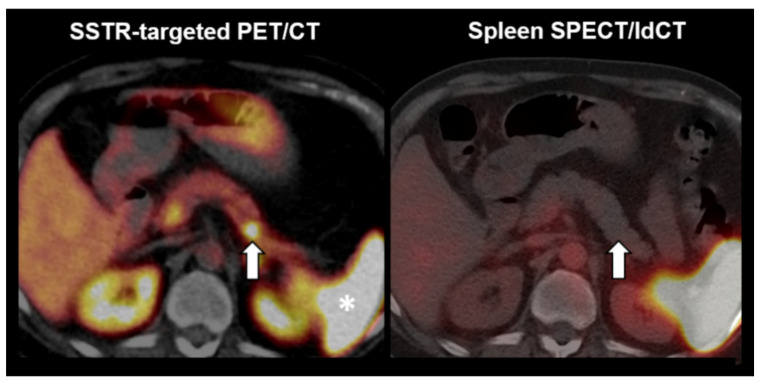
Example of a negative reading in spleen scintigraphy. (Left) Somatostatin receptor (SSTR)-targeted PET/CT. (Right) ^99m^Tc-labelled denatured red blood cells SPECT fused to low dose CT. Arrows = the suspected lesion. Asterisk = physiologic SSTR radioligand uptake in the spleen.
